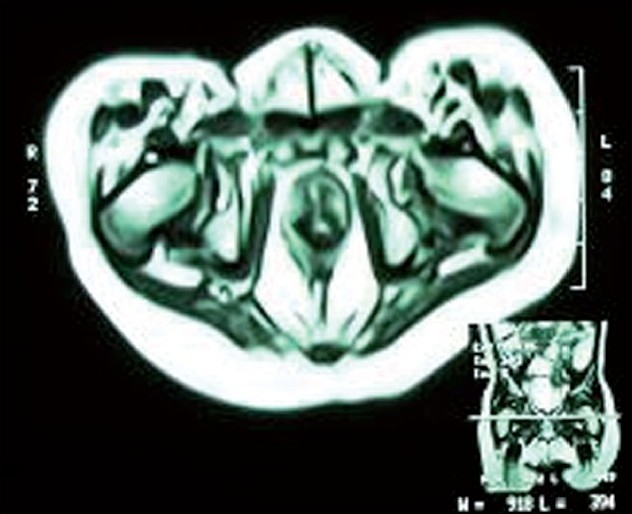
Postop MRI appearance of pelvis
